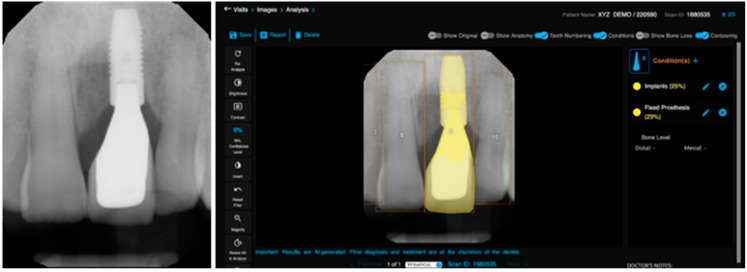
The automatic feature segmentation of the teeth numbering, implant, and fixed prosthesis detection in an anterior periapical radiograph.
